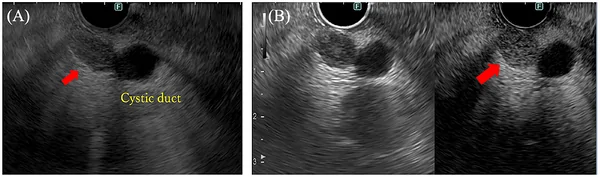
Endoscopic ultrasonographic findings. (A) Endoscopic ultrasound (EUS) demonstrated an 8‐mm well‐defined homogeneous hypoechoic lesion within the remnant cystic duct (red arrow). (B) Contrast‐enhanced EUS using perflubutane (Sonazoid; GE Healthcare, Tokyo, Japan) demonstrated marked enhancement of the lesion at 15 s after contrast injection (red arrow).
